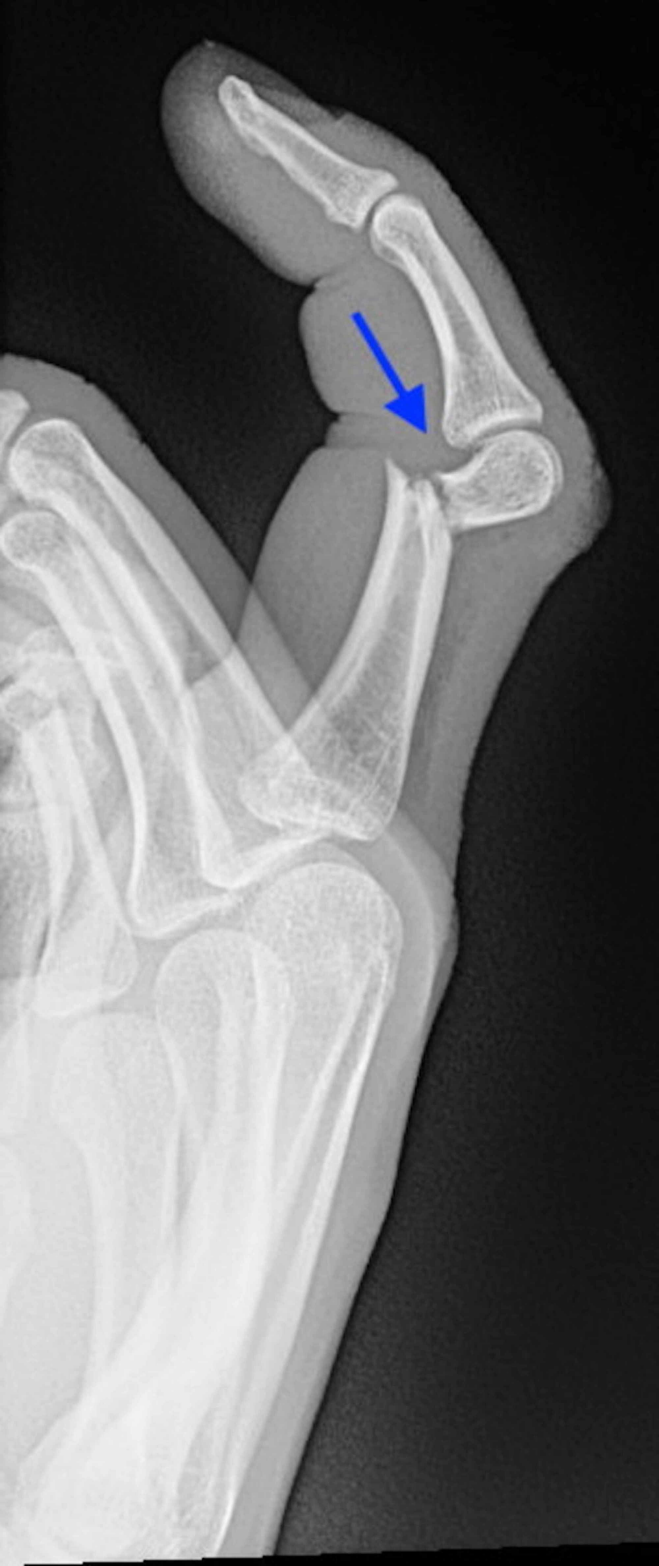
Lateral plain radiograph of the right index finger demonstrating fracture of the distal third of the proximal phalanx (arrow) with no bone-to-bone contact and intact articular surfaces
